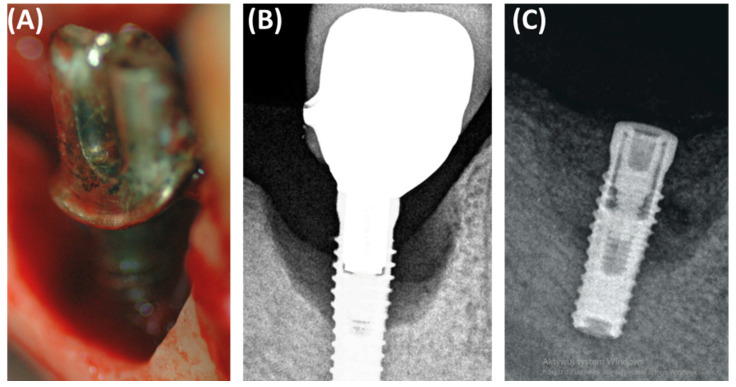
Images showing the defect resulting from periimplantitis: (A) clinical photo, (B) radiographic photo, (C) photo after defect restoration with bone substitute material.
